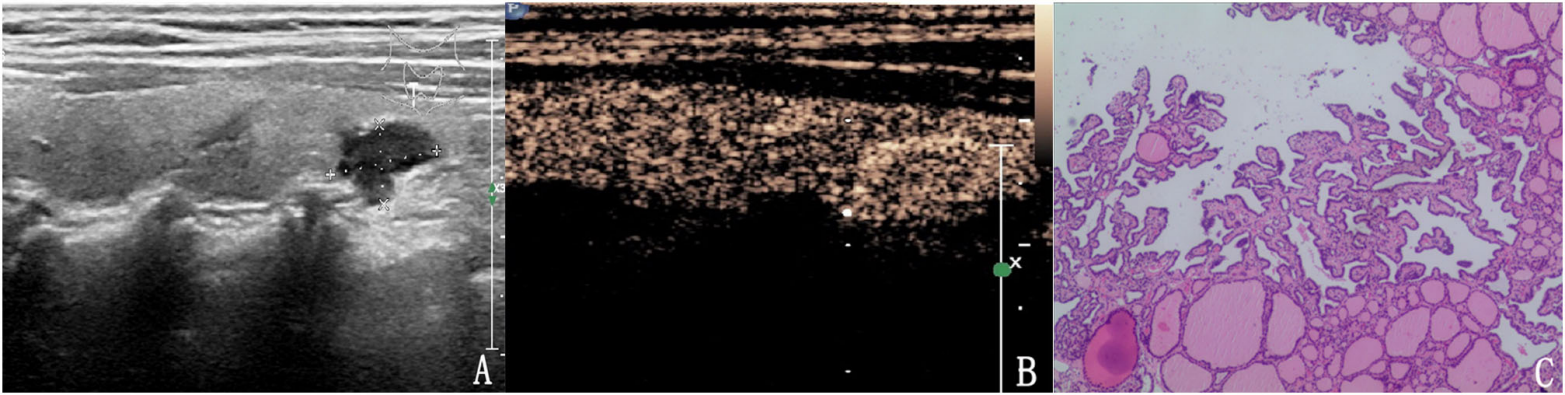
The case of a 64-year-old woman with a 10.6 × 6.7 × 5.9 mm solid mark hypo-echoic nodule in the right lobe of the thyroid. (A) Conventional two-dimensional image showing that the nodule had three malignant indicators (solid, mark hypo-echoic and irregular margin) and was classified with a TI-RADS score of 4b. (B) Ultrasound contrast image showing high enhancement. The modified version of the TI-RADS combined with CEUS returned a score of 4a, and the diagnosis was a benign nodule. (C) Pathological image of the lesion, a nodular goiter.
